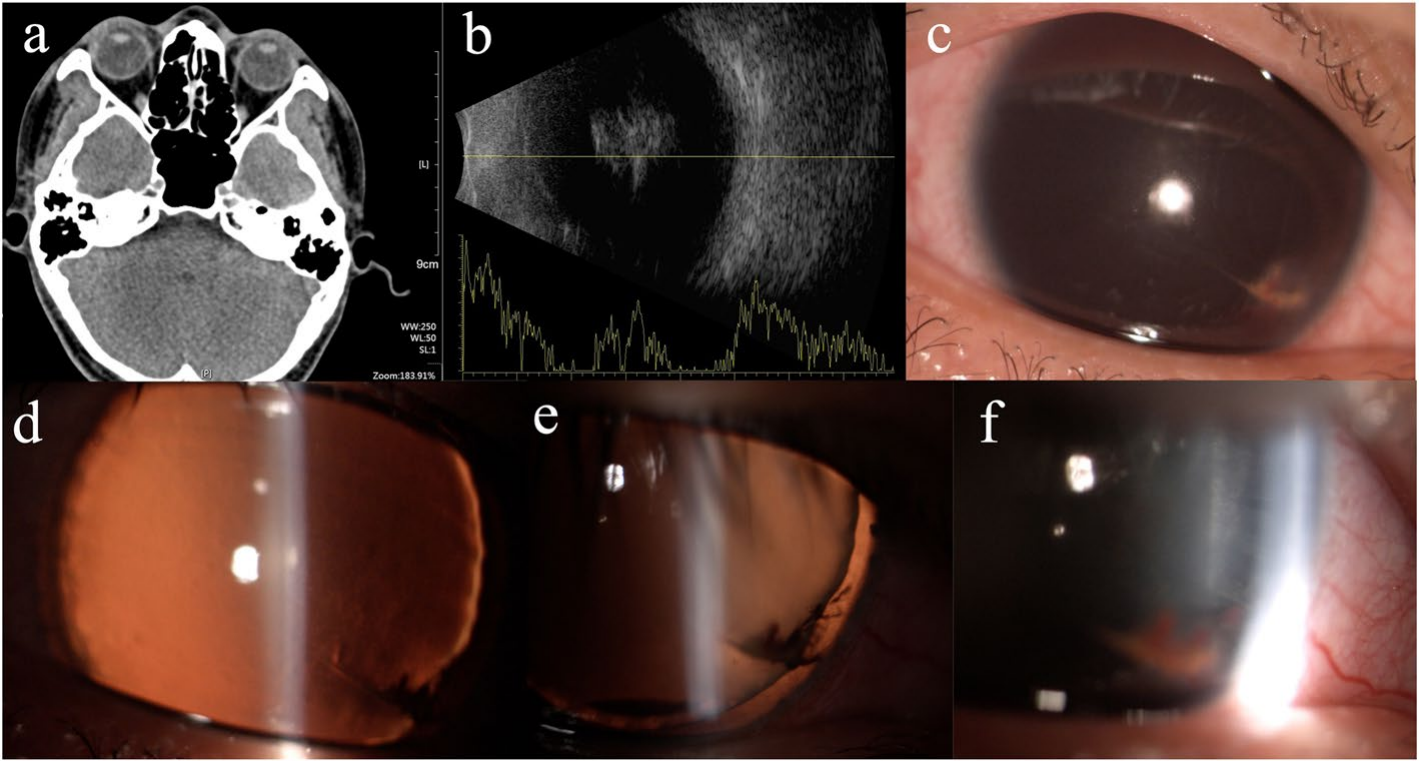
No intraocular foreign bodies were found on computed tomography scan a B-scan revealed mass opacity in the vitreous cavity 6 h after injured b 36 h after initial examination, a 2-mm full-thickness oblique laceration on the inferior-nasal aspect of the cornea c intact clear lens d, e zonules fibers f and complete loss of the iris are observed after resolution of the hyphema
